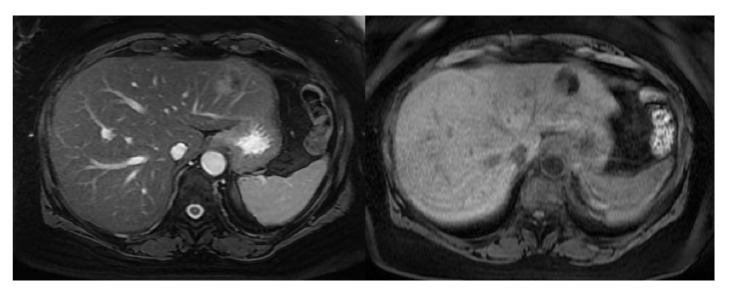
MRI*. FIESTA (right) and T1-weighted images (left) revealed a 3.1 x 3.4 x 2 cm mass in the left lateral lobe of the liver. The lesion is hypervascular with washout of contrast on delayed post-contrast enhanced sequences.
